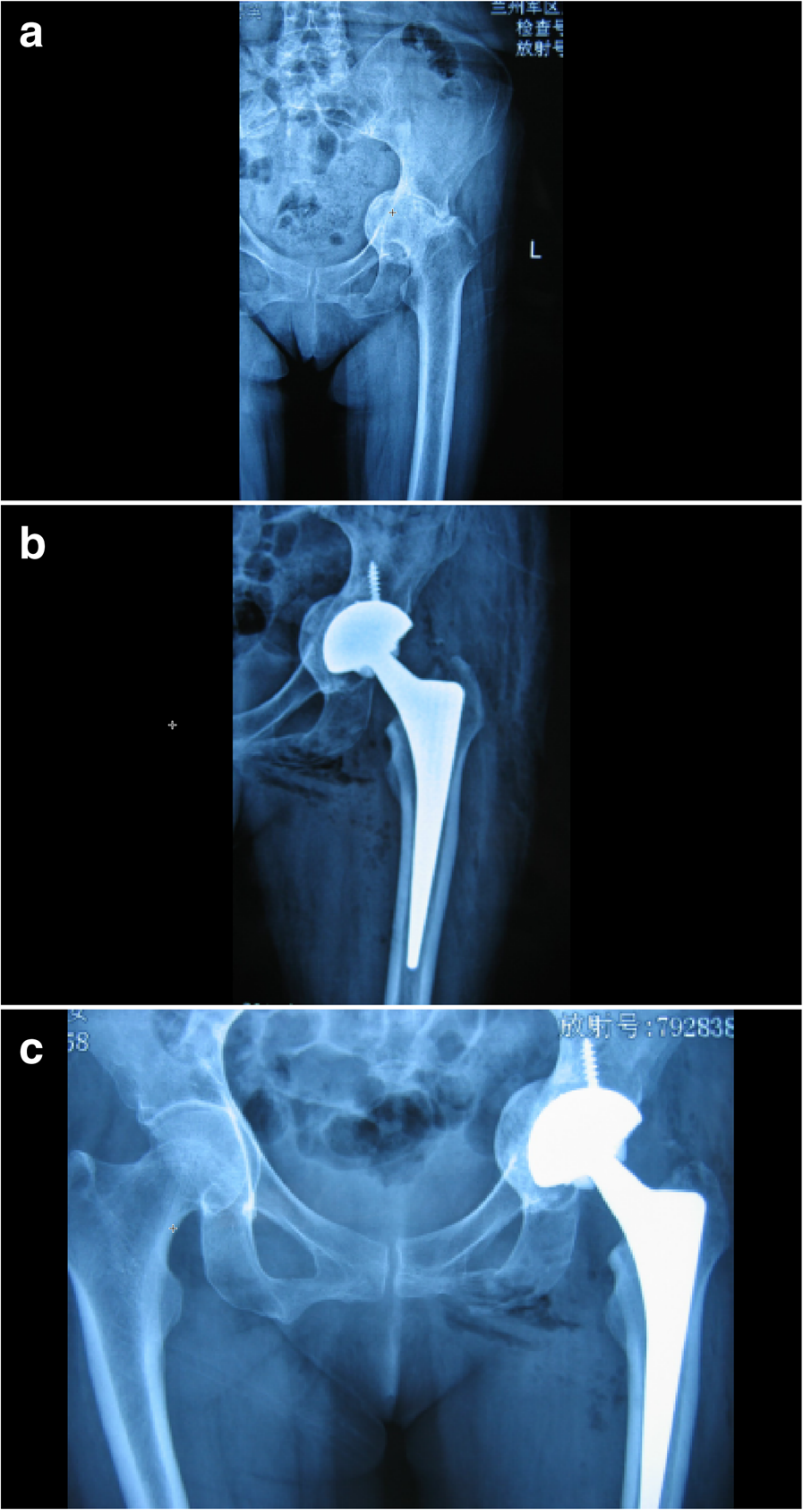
a Left hip exhibiting rheumatoid arthritis (RA) with an acetabular protrusion. The femoral head protruded inward over Nelaton’s line. The disease was of Sotello-Garza and Charnley type II. b Total hip arthroplasty (featuring placement of a porous tantalum acetabular cup; Zimmer) accompanied by acetabular reconstruction with autologous bone. An X-ray taken immediately after surgery revealed that the hip joint rotational center had returned to the normal location. The initial acetabular cup stability was good. c At the 5-year follow-up, an X-ray revealed complete bone graft healing, without bone resorption or acetabular loosening
